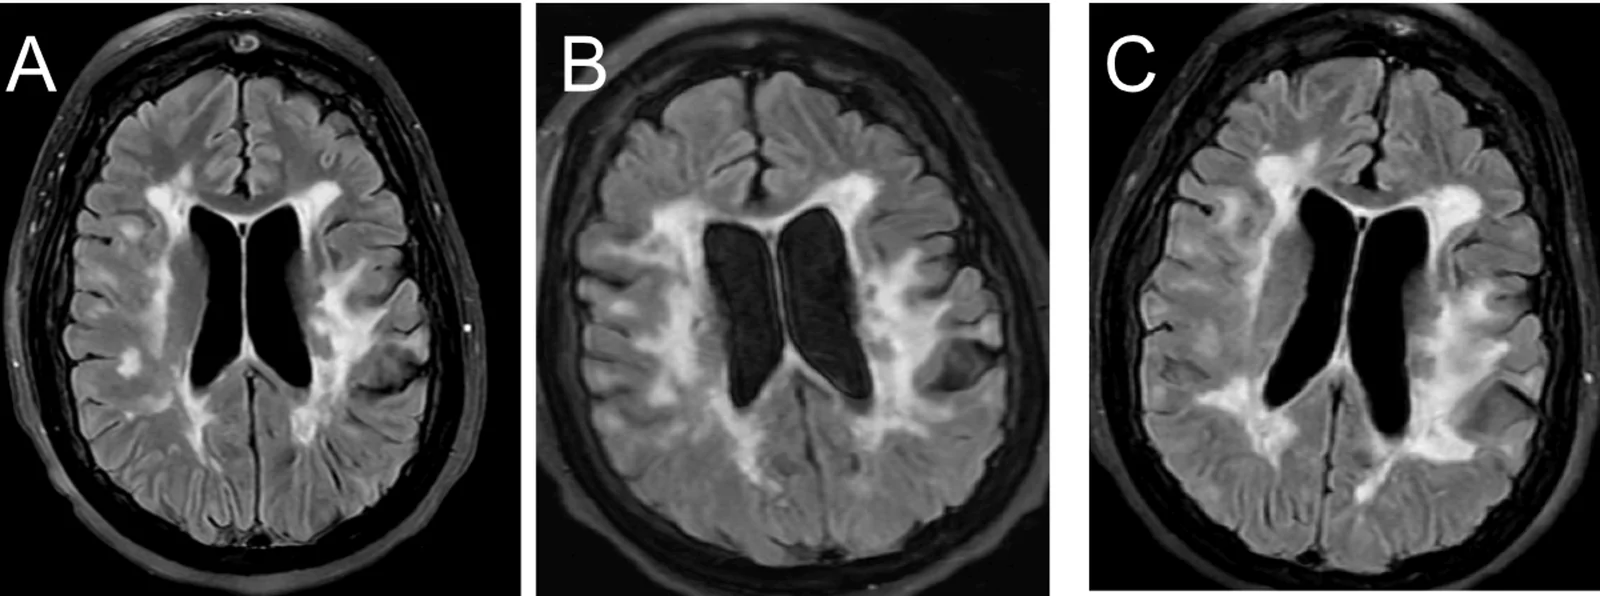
Representative axial T2-FLAIR brain MRI at three timepoints: (A) ~4 years before admission 1, (B) day 1, and (C) day 53. The distribution of confluent supratentorial periventricular and subcortical T2-FLAIR hyperintensities remained similar across examinations. The corresponding radiology reports documented no abnormal postcontrast enhancement or diffusion restriction, with no radiographic evidence of active demyelination. FLAIR: fluid-attenuated inversion recovery
